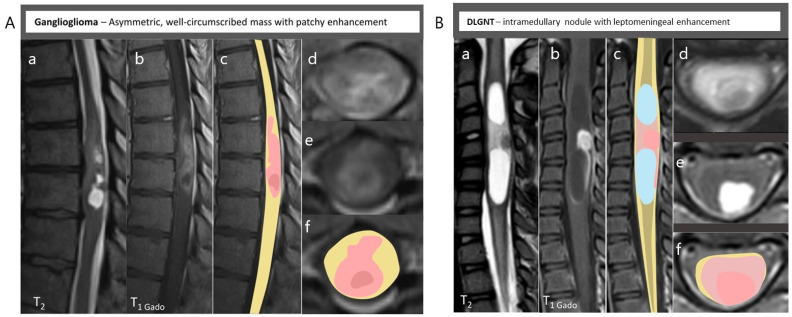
(A) Ganglioma: (a) Sagittal T2-weighted image showing asymmetric intramedullary lesion with intratumoral cysts; (b) Sagittal T1-contrast-enhanced image showing a mild enhancement; (c) Sagittal pictorial representation; (d) Axial T2-weighted image; (e) Axial T1-contrast-enhanced image; (f) Axial pictorial representation. (B) Diffuse leptomeningeal glioneural tumor: (a) Sagittal T2-weighted image heterogeneous isosignal lesion with hyposignal nodule, associated with syrinx and edema; (b) Sagittal T1-contrast-enhanced image showing nodular and subpial enhancement; (c) Sagittal pictorial representation; (d) Axial T2-weighted image; (e) Axial T1-contrast-enhanced image showing a high enhancement of the nodule; (f) Axial pictorial representation.
